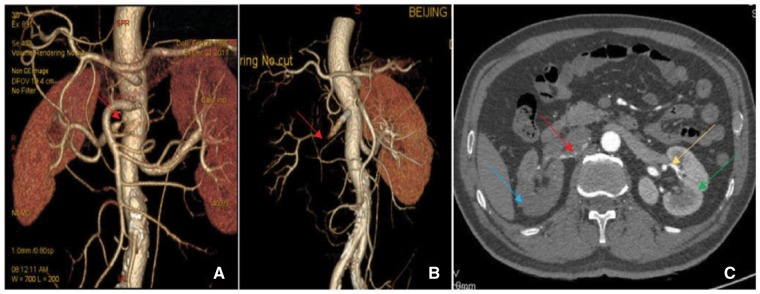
Preoperative renal artery computed tomography angiography shows that the right renal artery stenosis (red arrow) was 95% (*A*). Two days after renal artery stenting, reconstruction of renal artery computed tomography angiography showed the lumen of the middle part of the right renal artery was occluded (red arrow) and right renal perfusion was not restored (*B*). The transverse section highlights a long linear filling defect of the right renal artery (red arrow) with full filling of the left renal artery (yellow arrow) and no filling with contrast media in the right renal cortex (blue arrow) with full filling with contrast media in the left renal cortex (green arrow) (*C*).
